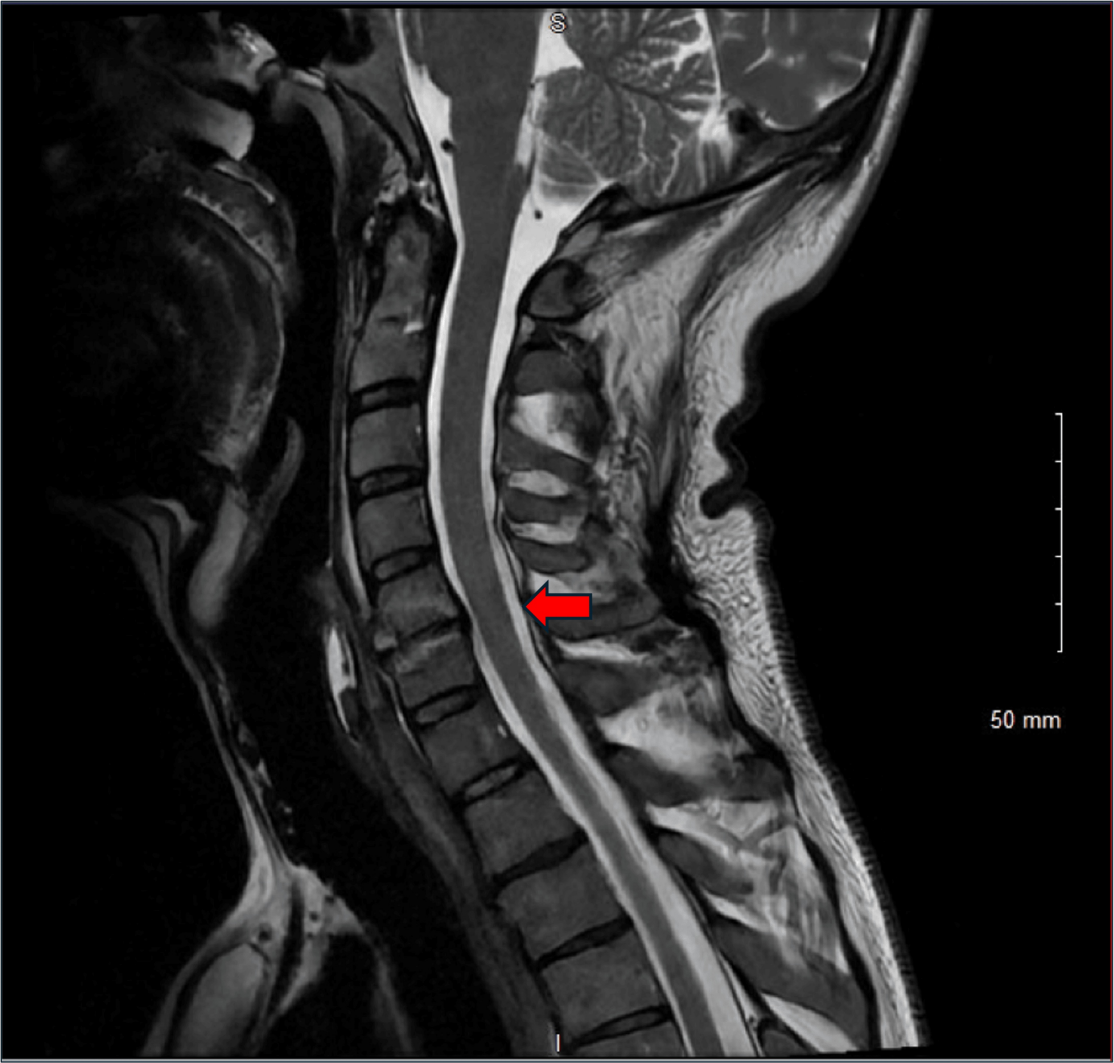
Magnetic resonance imaging (MRI) of the cervical spine showing infective discitis at C5–C6 (red arrow).
